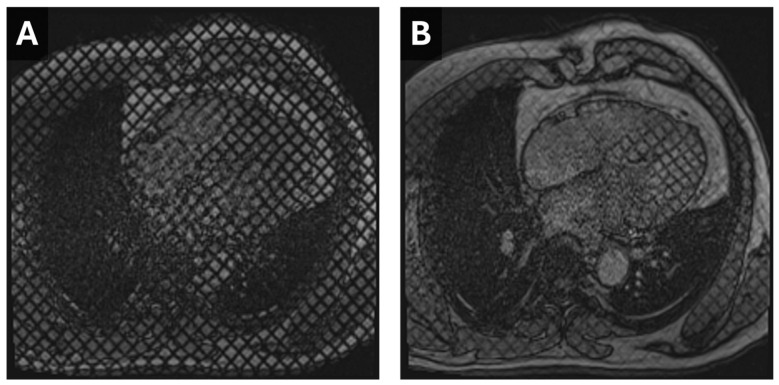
Cardiac MR Strain Evaluation. Static horizontal long-axis diastolic (A) and systolic (B) grid-tagged images from a cardiac MRI. Deformation of the grid as the myocardium contracts can be used to estimate strain.
